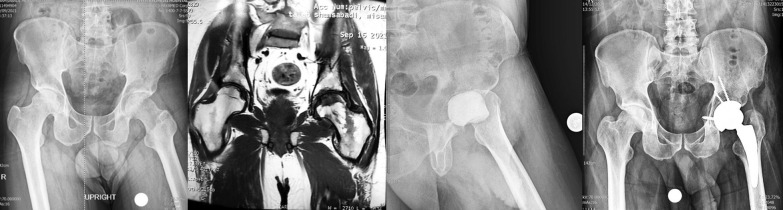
a Case one, preoperative X-ray, left hip involvement. b MRI of the hips shows left side inflammation and cartilage destruction. c Early post-op after first stage of arthroplasty. d) Post-op radiography, after 2nd stage
